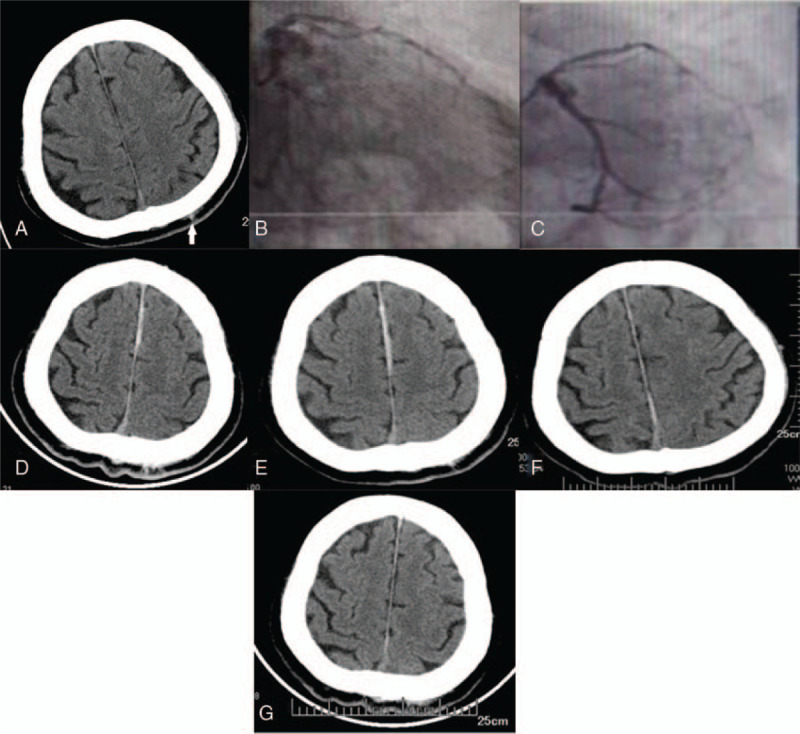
A 71-year-old man suddenly lost consciousness and fainted 2 hours prior to admission with a history of trauma to his head. The patient experienced a felt headache and discomfort upon waking. (A) Initial head CT revealed a small, left parietal subcranial hematoma (arrow). (B) Left coronary angiography finding showed that the wall of the left anterior descending artery was irregular, the proximal segment was severely stenosed, the left circumflex branch was thick, the wall was irregular, and the middle and distal segments showed moderate stenosis. (C) After percutaneous coronary intervention, left anterior descending artery stent expansion was satisfactory without residual stenosis. (D) Head CT following percutaneous coronary intervention showed that the anterior longitudinal fissure of the brain exhibited a band-like, high density with uneven thickness (about 2–5 mm), and the CT value was about 59–70 HU, with an average of 65 HU. The edge of the falx side of the brain was straight, smooth, and sharp, and the edge of the parenchymal side was clear without obvious edema or a space-occupying effect. (E) The high density range of the anterior longitudinal fissure increased at 5 hours. (F) At 22 hours, the lesions were basically absorbed. (G) Head CT re-examination suggested that the banded high density in the cerebral hemisphere was completely absorbed 2 days later.
